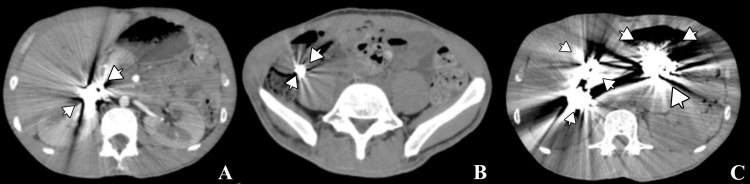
Abdominal computed tomography scan showing metallic foreign bodies (arrows) in the gastric chamber, small intestine, and colon. (A) Metallic foreign bodies in the duodenum. (B) Metallic foreign bodies in the colon. (C) Metallic foreign bodies in the gastric and duodenal chambers.
